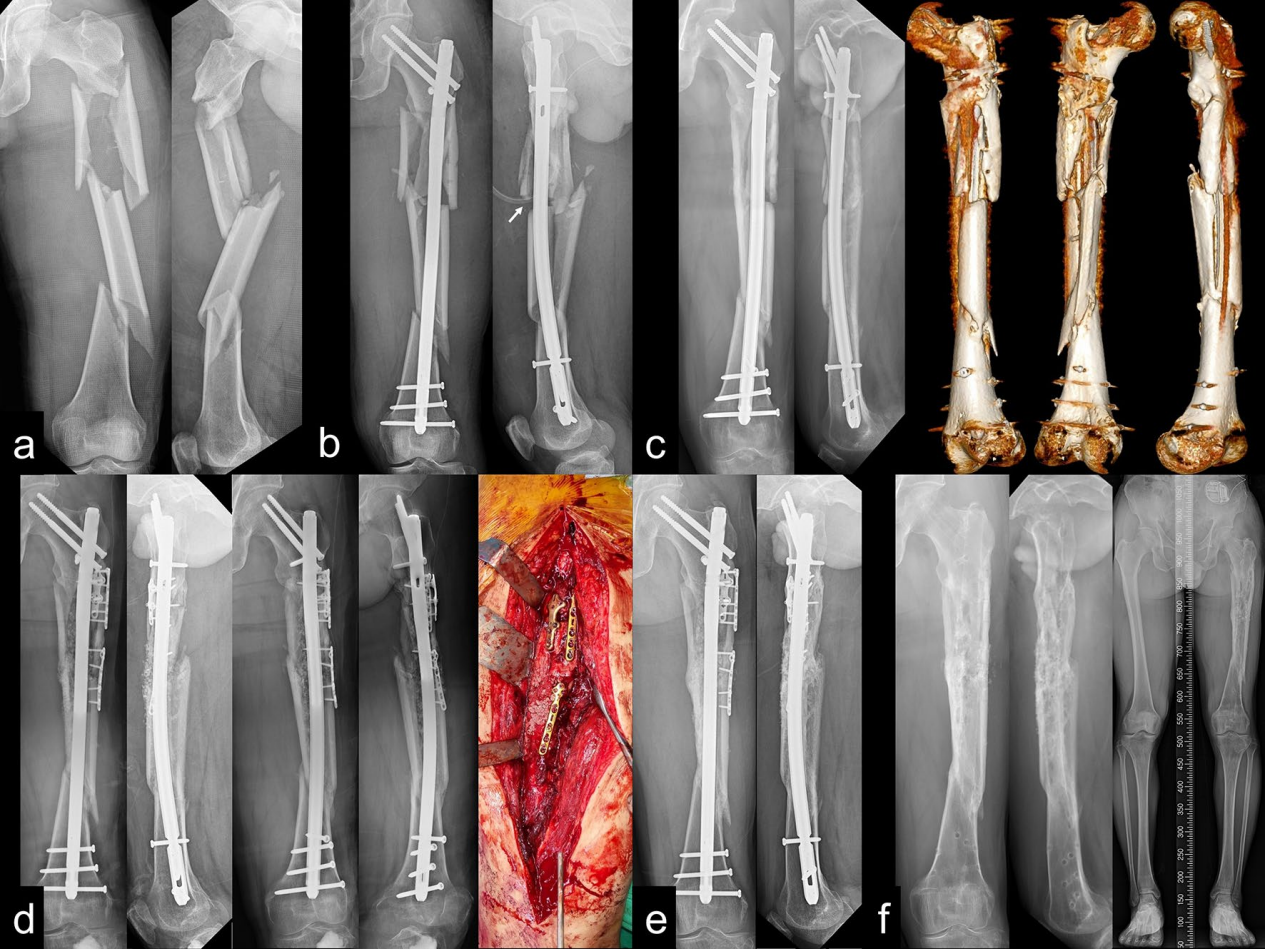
(a) A 51-year-old male patient was diagnosed with a femoral shaft fragmentary segmental fracture (AO/OTA 32C3) on the left side. (b) Reamed antegrade intra-medullary femoral nailing was performed using two reconstruction proximal interlocking screws, one proximal blocking screw and four distal interlocking screws. The exposed nail length was measured as 28 mm in immediate post-operative radiographs. (c) Nine months after surgery, the patient developed non-union. Three-dimensional reconstruction of computed tomography images showed the nail partially exposed owing to cortical bone defects where comminution was initially located. (d) On diagnosis of non-union, the patient underwent osteotomy and repositioning of vitalised cortical bone fixed with small fragment-specific plates, followed by autogenous and bone substitute grafts to fill the cortical bone defect. (e) Non-union completely healed 13 months following revision surgery. (f) X-ray image showing a fully consolidated and united femoral shaft fragmentary segmental fracture after implant removal surgery. There was no significant angular deformity or shortening with acceptable alignment.
